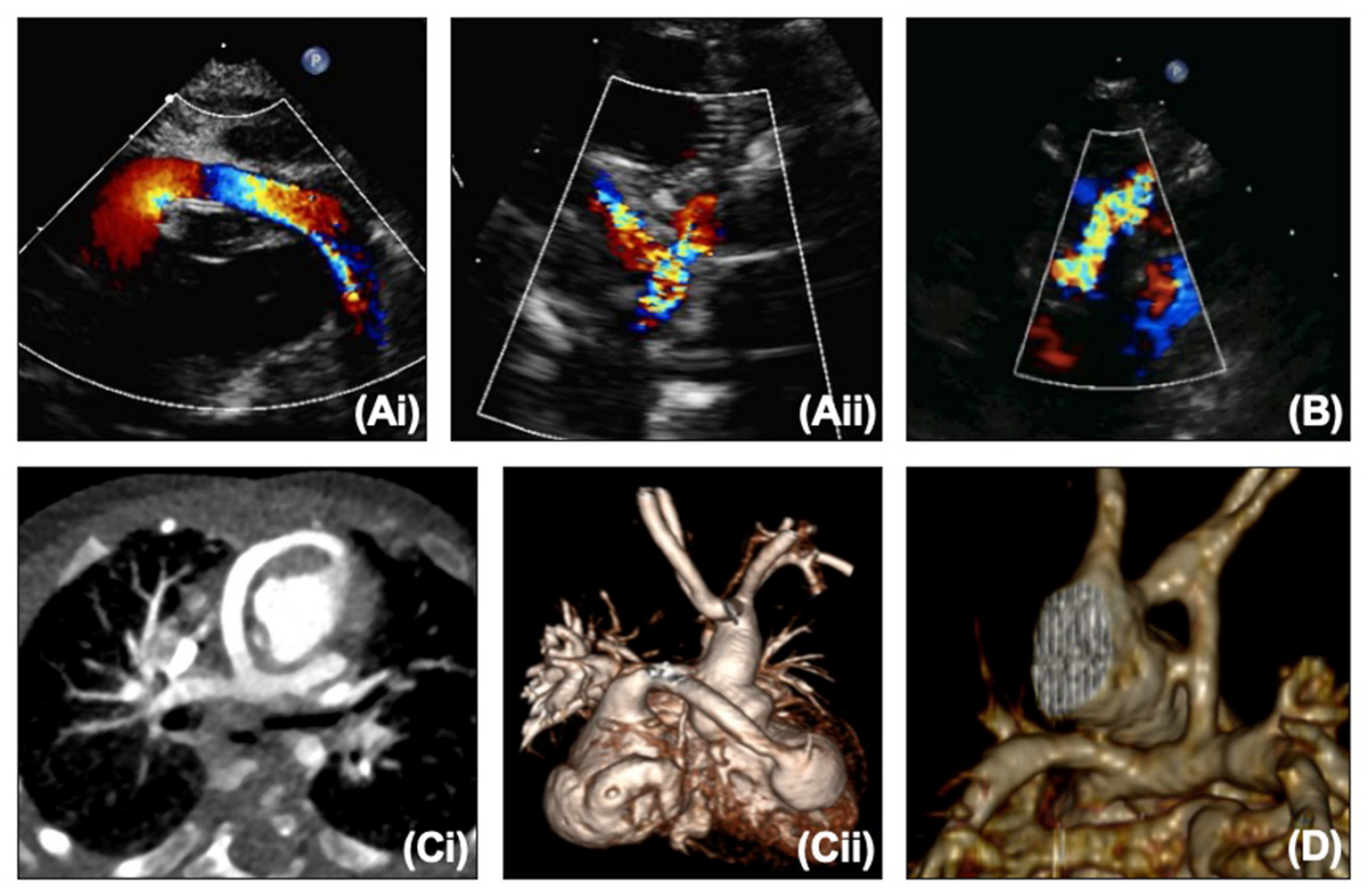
(A,i) Proximal and (A,ii) distal right ventricle to pulmonary artery conduit on echocardiography. (B) Modified Blalock-Taussig-Thomas shunt on echocardiography. (C,i,ii) Right ventricle to pulmonary artery conduit on CT. (D) Modified Blalock-Taussig-Thomas shunt on CT.
